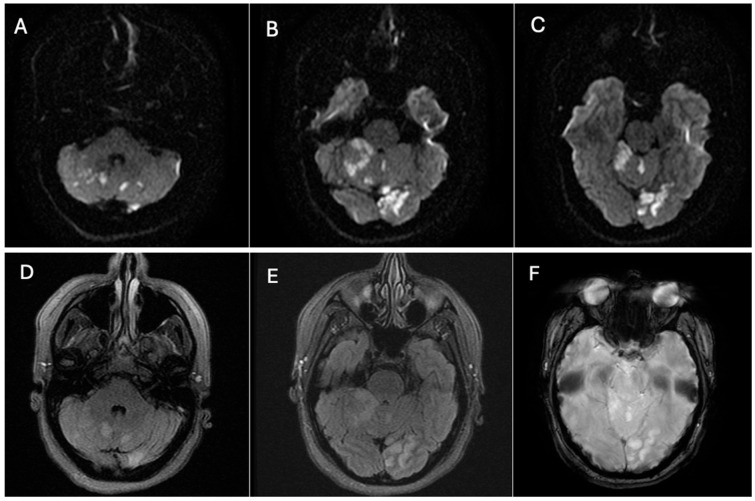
Brain magnetic resonance imaging (MRI): (A–C) Axial diffusion-weighted imaging (DWI) sequence (TR/TE = 3600/90 ms), showing multiple focal lesions in the left occipital lobe, left cerebellar peduncle, and both cerebellar hemispheres, more prominent on the right side. These lesions demonstrate marked diffusion restriction, consistent with acute embolic infarction. (D,E) Axial T2-weighted FLAIR sequence (TR/TE = 9000/120 ms), showing corresponding hyperintense signal abnormalities. (F) Axial gradient-echo T2* sequence (TR/TE = 700/20 ms), demonstrating no evidence of intracranial hemorrhage. Source: patient’s medical record, with prior authorization.
